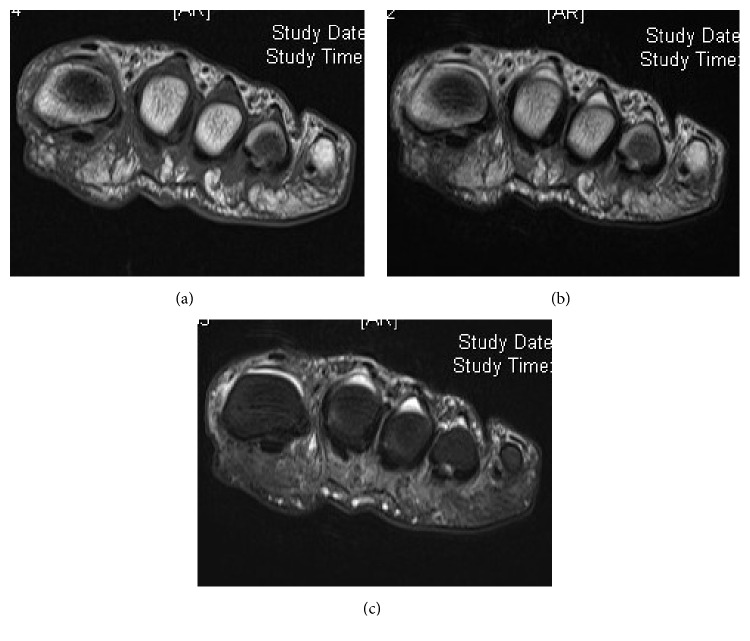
Magnetic resonance images of the distal metatarsals showing an upside-down bulbous-shaped mass with low-signal intensity in T1-weighted (a), T2-weighted (b), and short-tau inversion recovery image (c) extending dorsally between the second and third metatarsal heads.
